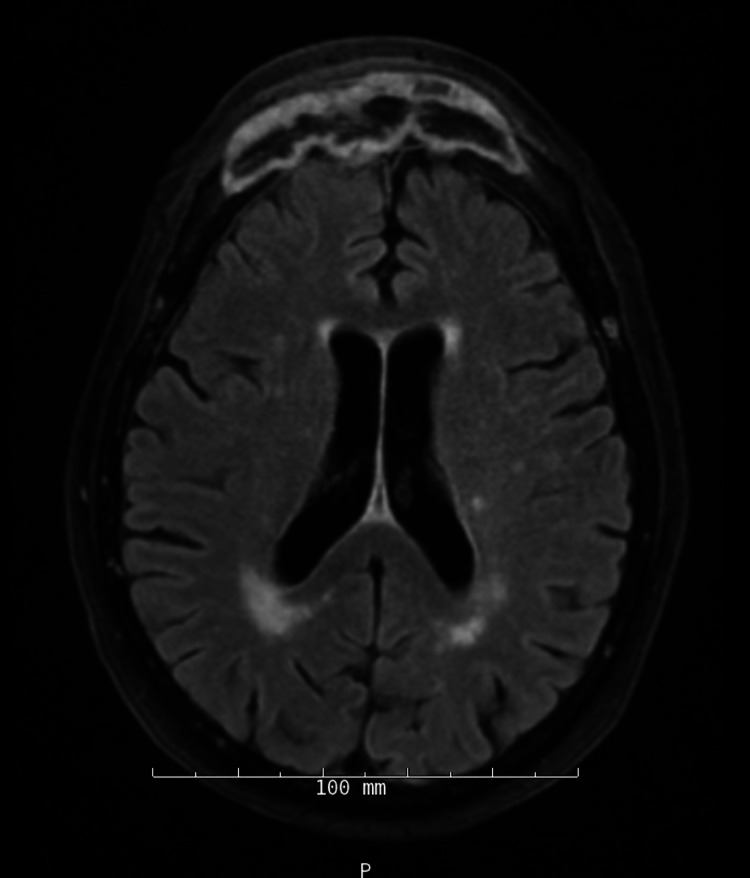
T2 Axial Flair MRI Brain With Persistent Frontal Sinusitis
